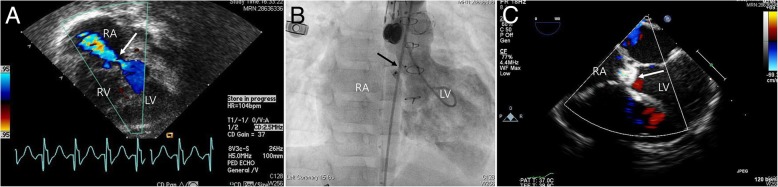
Device closure of Gerbode postoperative VSD with Amplatzer duct occlude I. a Gerbode shunt (LV-RA) was shown on transthoracic echocardiography and color Doppler (white arrow). b LV angiography showed Amplatzer duct occlude I in a good position after implantation (black arrow). c Transthoracic echocardiography showed successful closure of Gerbode shunt with device (white arrow). LV, left ventricle, AO, aorta, RV, right ventricle, RA, right atrium, VSD, ventricular septal defect
